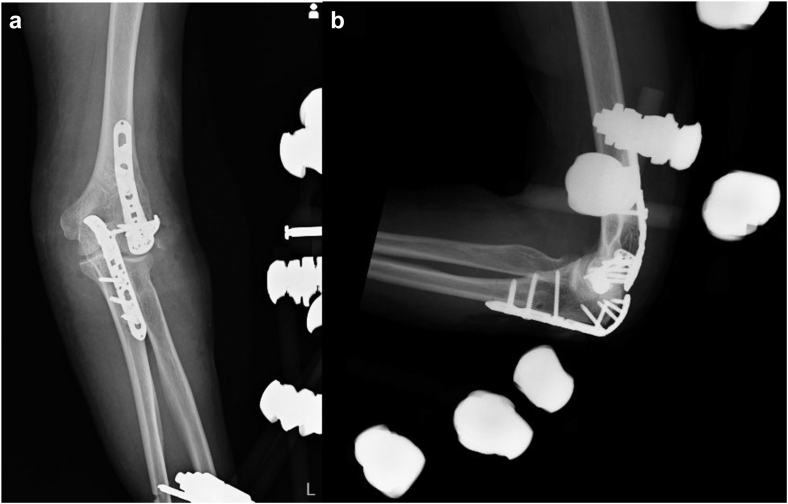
Postoperative plain radiographs. (a) Anteroposterior radiograph, (b) lateral radiograph.
